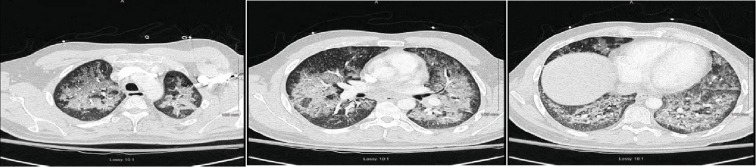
Chest CT showing bilateral airspace disease.
